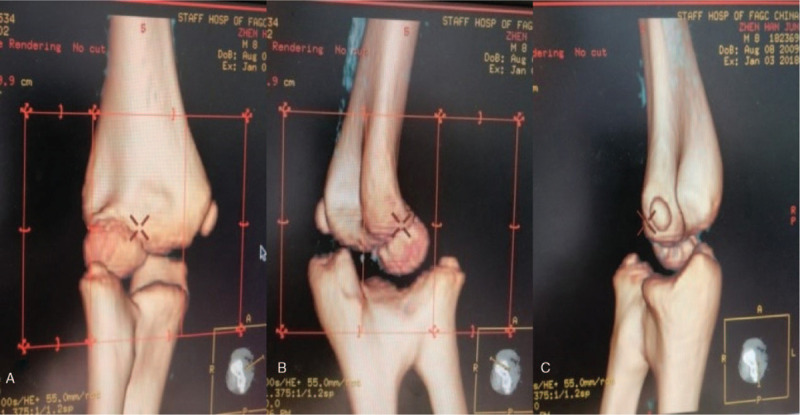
(A) Preoperative 3-dimensional computed tomography (3D CT) scan of the anteroposterior elbow. (B) Preoperative 3D CT scan of the oblique elbow. (C) Preoperative 3D CT scan of the lateral elbow.
